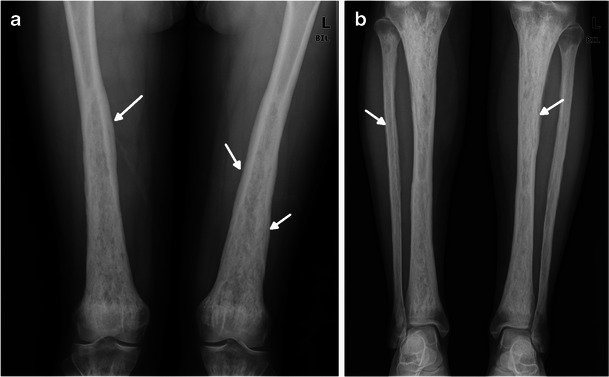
a AP radiographs of the distal femora in a 39-year-old female. Bone involvement is bilateral and symmetric with diaphyseal and metaphyseal heterogeneous osteosclerosis. Distal femora are enlarged, and periostitis is visible as a wavy contour (arrows) of the outer cortex. Corticomedullary margins in the diaphysis are blurred. b AP radiograph of two legs in the same patient. Both tibiae and peronei are involved. Corticomedullary margins in the diaphysis are blurred, and the marrow cavity is obliterated. Periostitis is also present (arrows)
